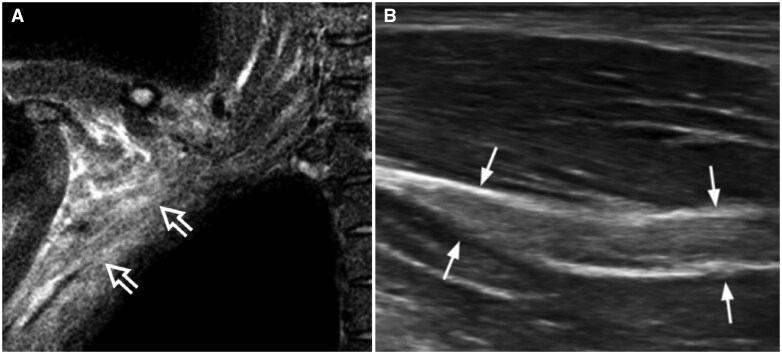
27-year-old man with arm weakness following traction injury. (A) T2-weighted fat-suppressed MR image shows severe oedema in the subcoracoid and axillary regions (open arrows) but no specific nerve injury. (B) Longitudinal ultrasound, on the following day, shows severe musculocutaneous nerve swelling (arrows) consistent with a severe traction injury.
